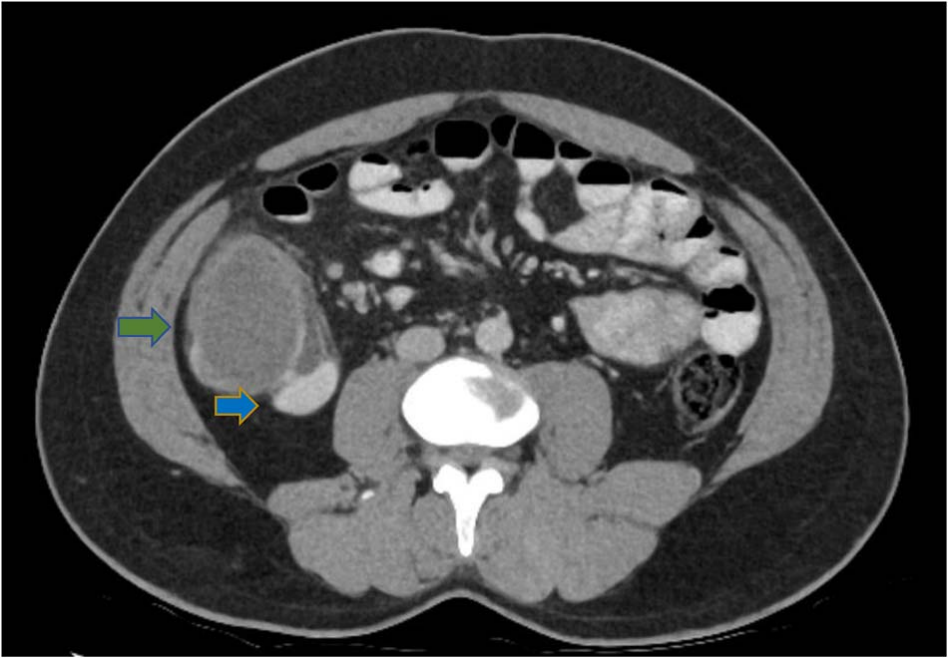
Axial CT abdomen demonstrating a cystic submucosal lesion at the right colon (green arrow). There is involvement of the colonic lumen but no obstruction with oral contrasting passing through the lumen (blue arrow).
